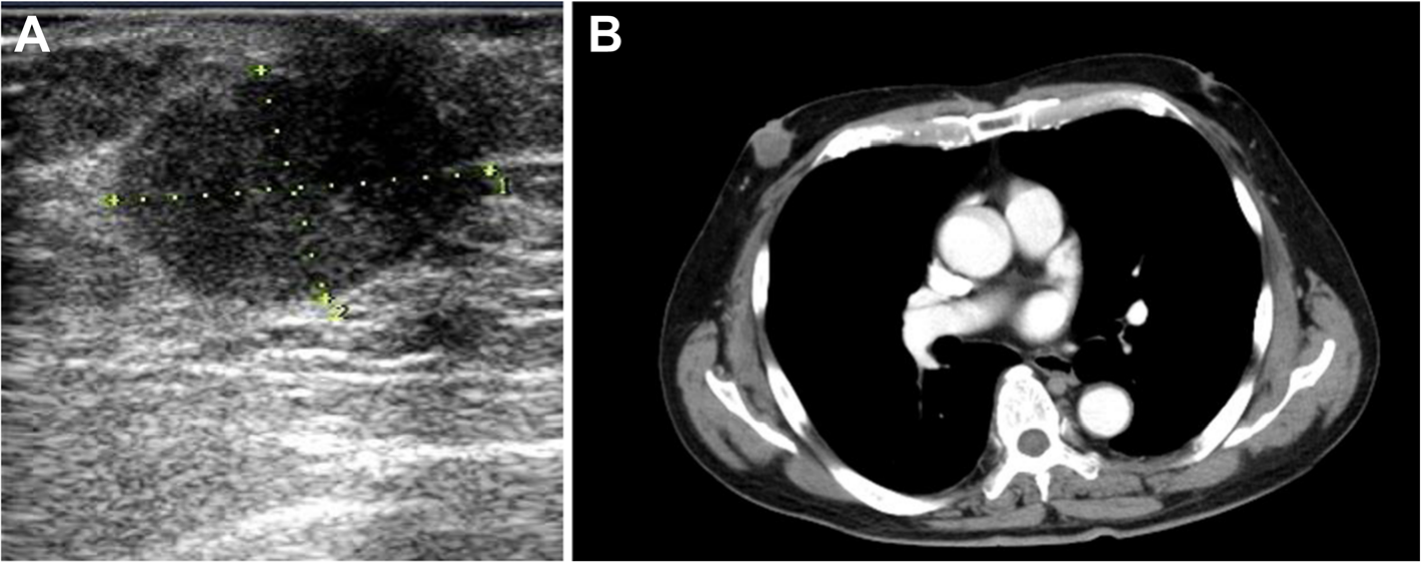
a A preoperative US scan showing a round mass of 24 mm in diameter in the right subareolar area. b A preoperative chest CT scan showing a round tumor of 24 mm in diameter in the right subareolar area
